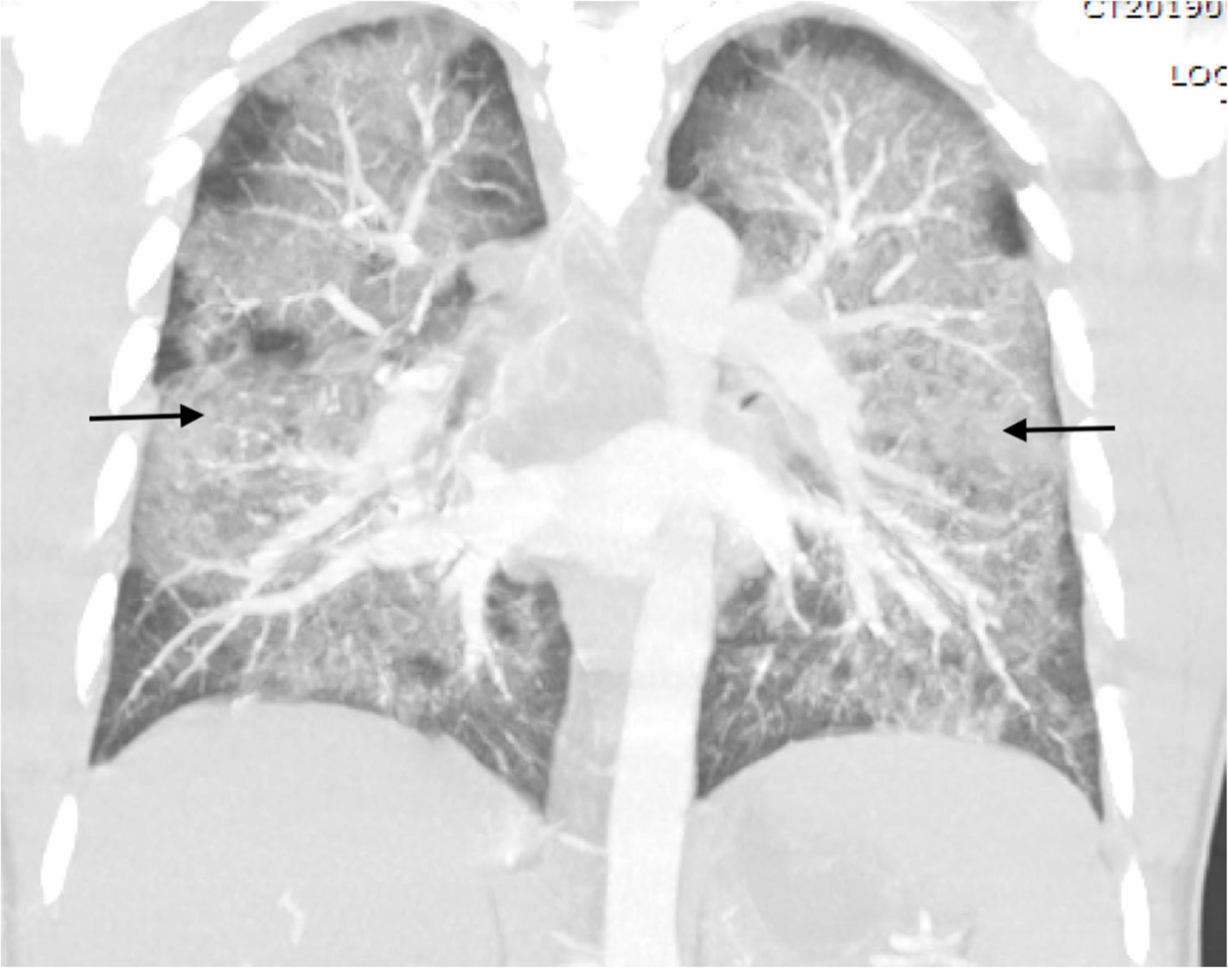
Sagittal view computed tomographic scan further demonstrating diffuse central predominant interstitial opacities (*black arrows*)
